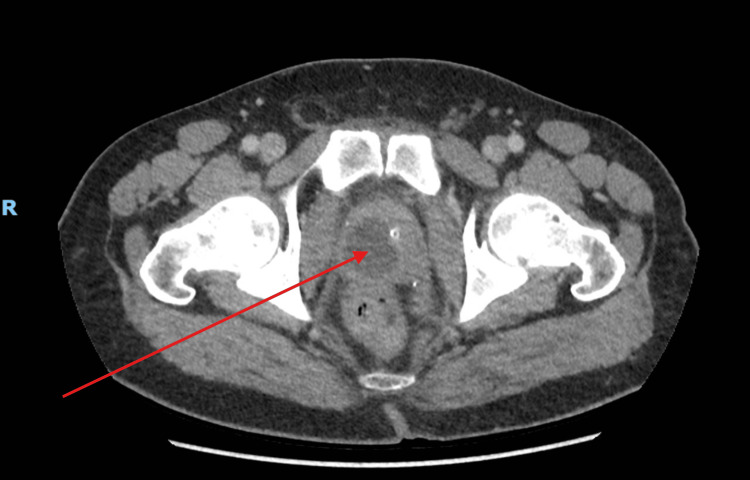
CT of the abdomen and pelvis on the day of admission (day zero). The red arrow points to the prostate abscess. The letter R depicts the right-hand side of the patient.
